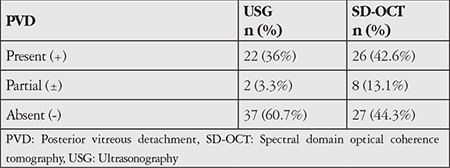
Presence of posterior vitreous detachment according to spectral domain optical coherence tomography and ultrasonography data
